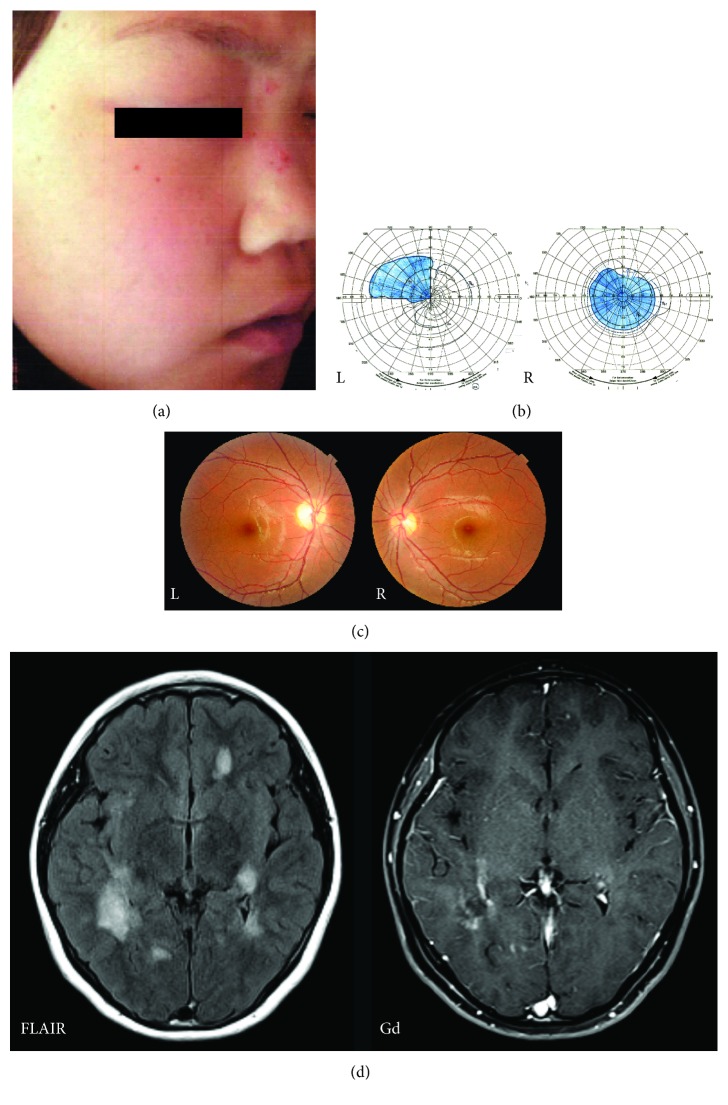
Clinical findings. (a) Skin rashes over the nasal sidewall and peripheral facial palsy on the right side 3 months before admission. (b) A visual field test on admission identified a central scotoma in the right eye and quadrantanopia in the left (L) eye. (c) The optic disc was atrophied and pale in the right (R) eye; there were no abnormal findings in the left eye. (d) Brain magnetic resonance imaging (MRI) on admission. Fluid-attenuated inversion recovery (FLAIR; left) imaging demonstrated multiple hyperintense periventricular deep white matter and juxtacortical lesions, some of which exhibited an open ring pattern on T1-weighted imaging after gadolinium (Gd) enhancement (right).
